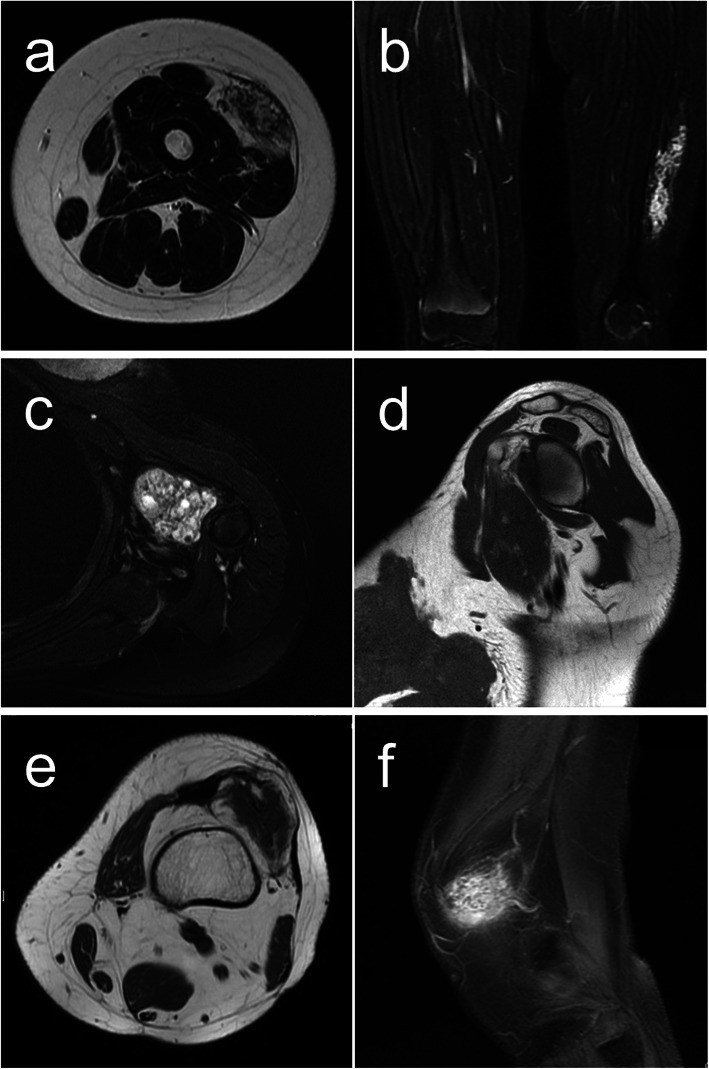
Magnetic resonance imaging (MRI). Axial T2-weighted MRI (a) and coronal fat-saturated enhanced T1-weighted MRI (b) of case 1. Axial fat-saturated T2-weighted MRI (c) and sagittal T1-weighted MRI (d) of case 2. Axial T1-weighted MRI (e) and sagittal fat-saturated T2-weighted MRI (f) of case 3
